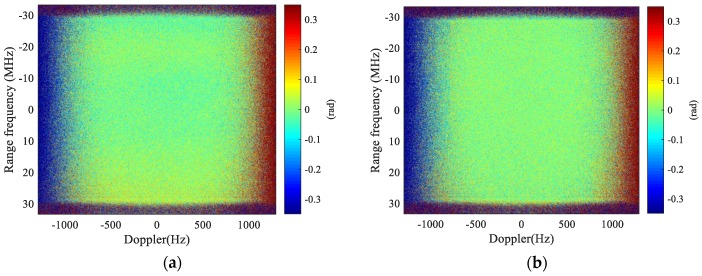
Phase imbalances in the 2-D spectral domain before and after imbalance correction (calibration bandwidths: 60 MHz in range and 1.58 kHz in Doppler): (a) before imbalance correction; and (b) after imbalance correction.
